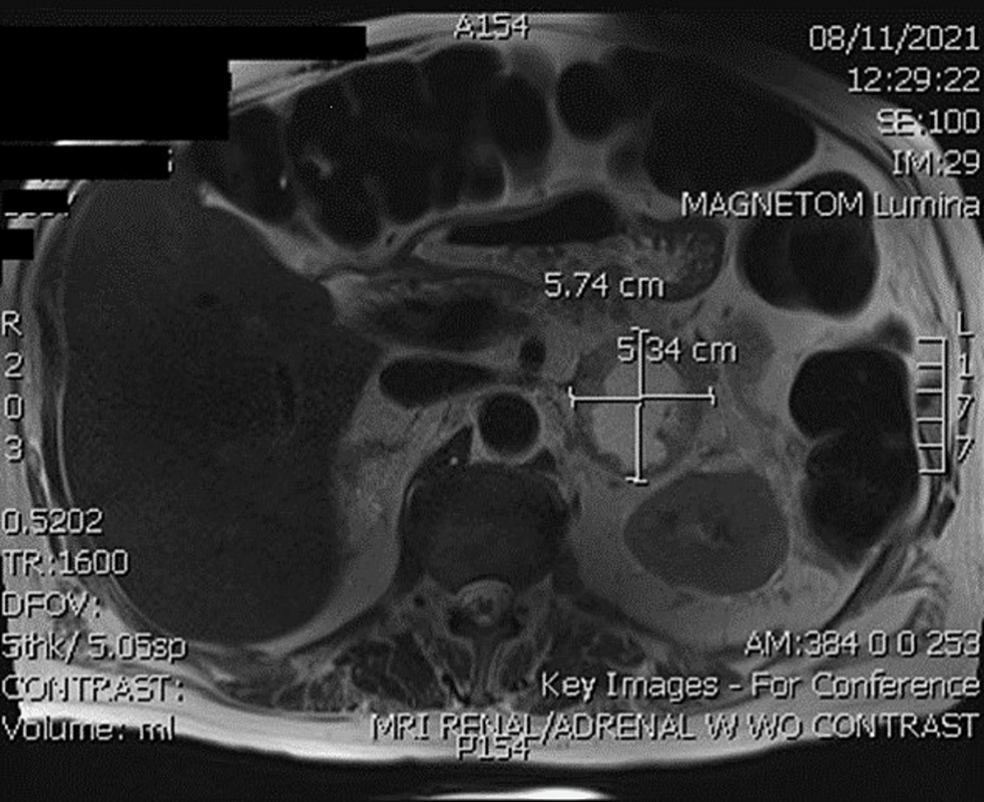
Magnetic resonance imaging transverse view. Magnetic resonance transverse view shows a 5.74 cm × 5.34 cm left adrenal mass.
